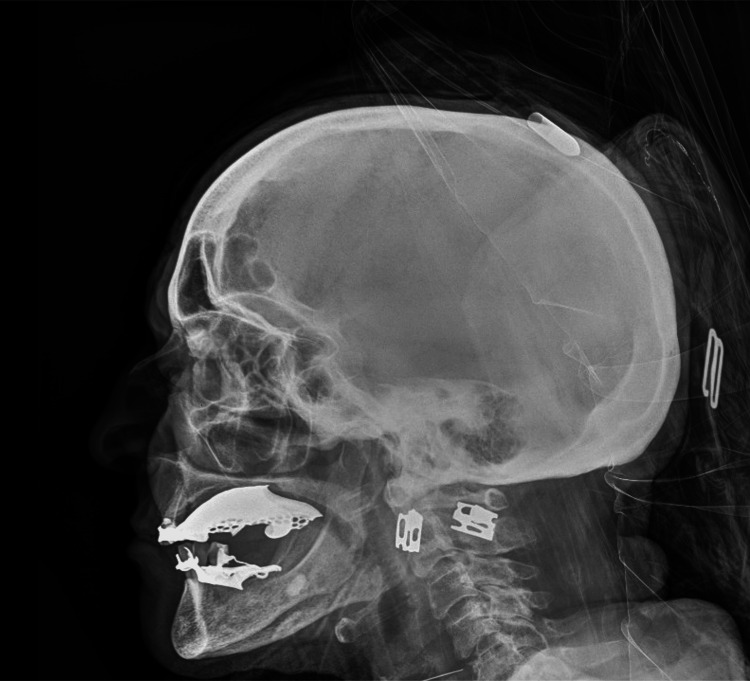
Lateral cranial X-ray. The arrow tip seems apparently lodged between the outer and inner cortical tables of the skull, without evidence of intracranial penetration.
